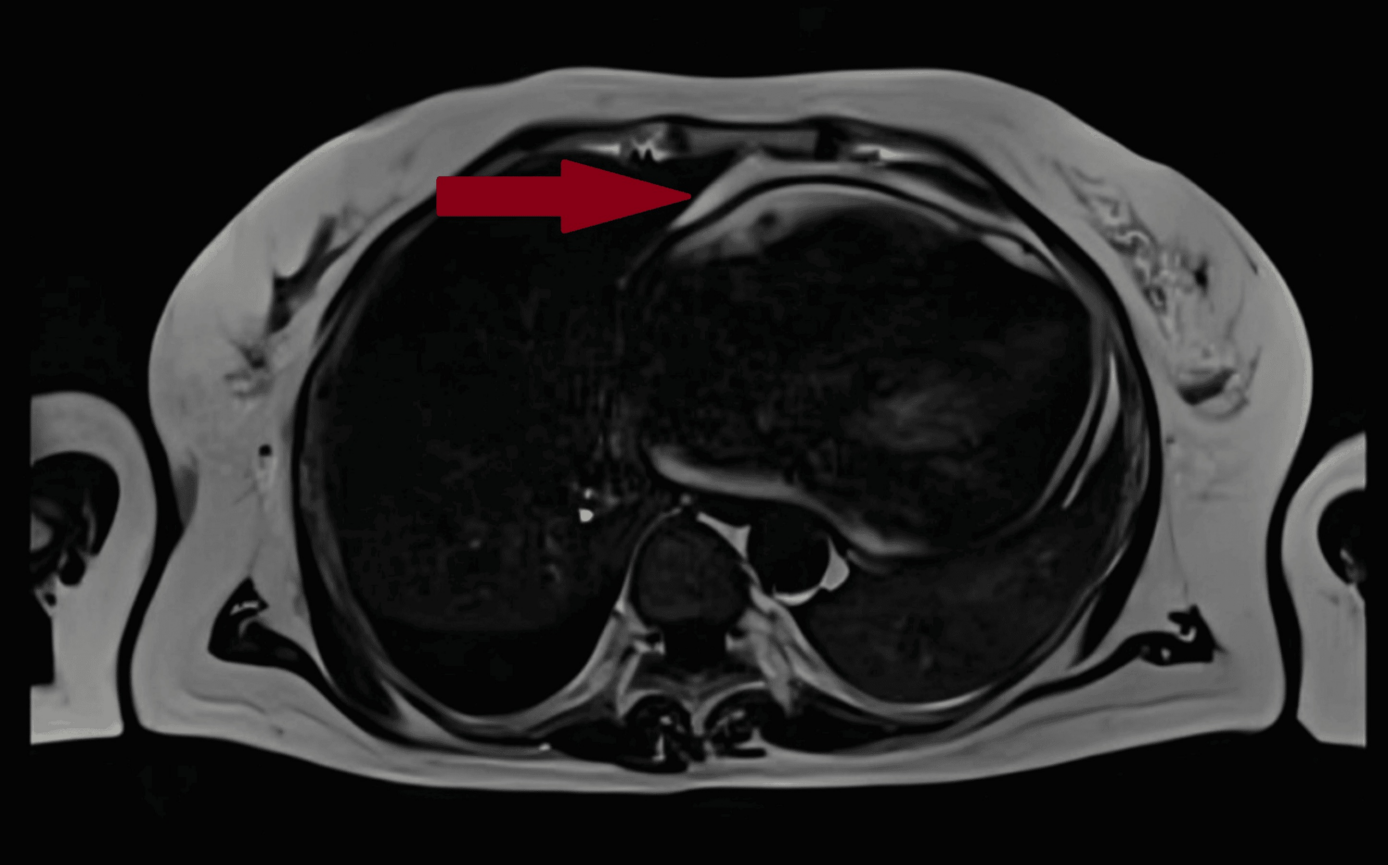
Cardiac MRI T1-weighted sequence. Thickening of the pericardium is seen. MRI: magnetic resonance imaging
